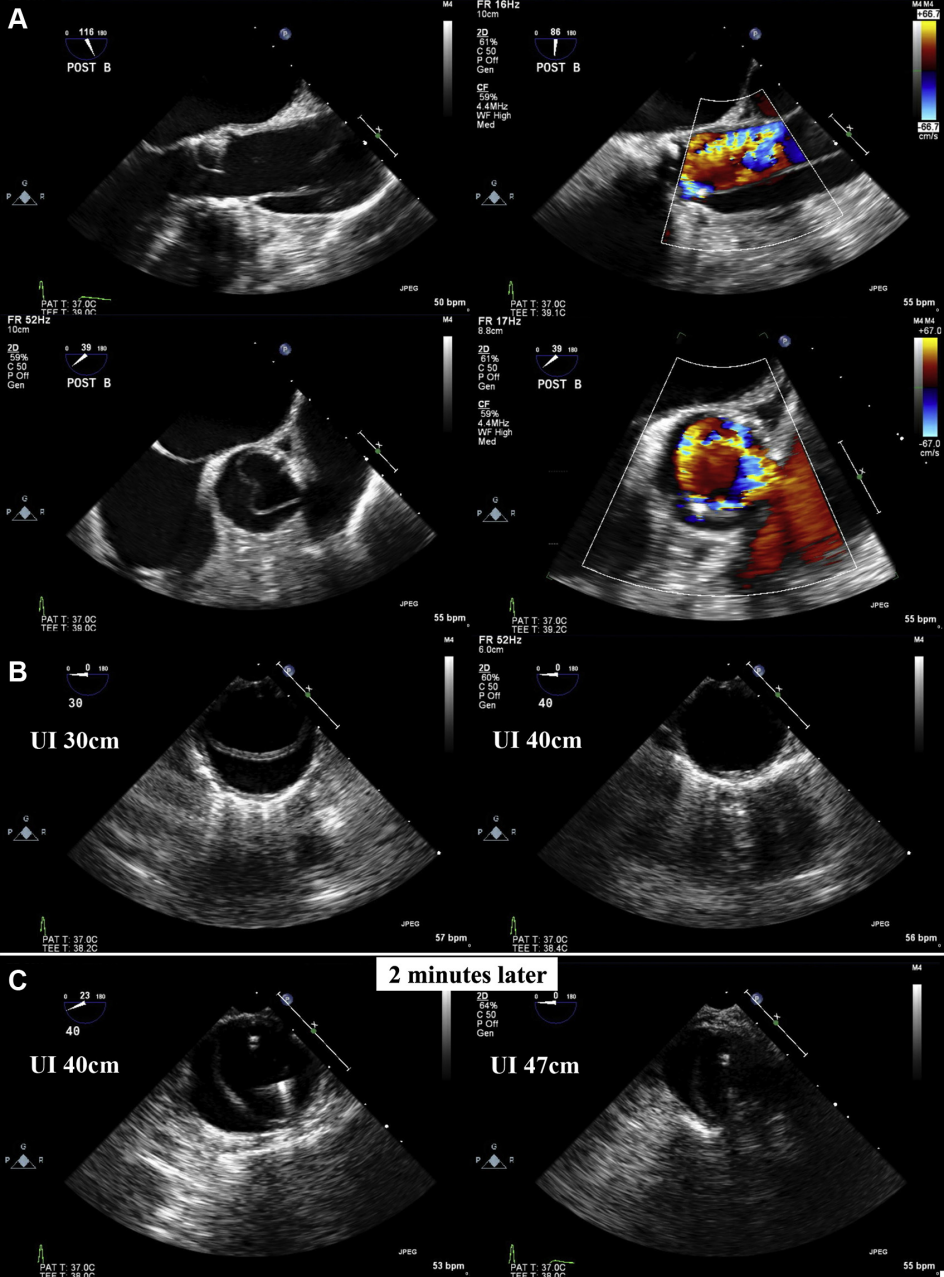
Transthoracic Echocardiography Images Immediately After Transthoracic Aortic Valve Replacement Transthoracic echocardiography images show the type A aortic dissection originating from the aortic root (A) and extending up to the descending thoracic aorta (B). Further distal propagation of the type A aortic dissection up to the abdominal aorta can be observed (C). UI = upper incisor.
